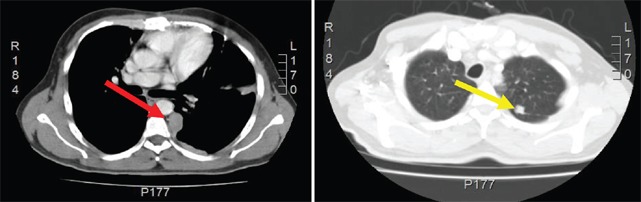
Computed Tomography of the chest shows left pleural nodular thickening predominantly in costophrenic recesses, identifying large tumors at this level (red arrow). Multiple left juxta-cisural nodules up to 13 mm (yellow arrow).
